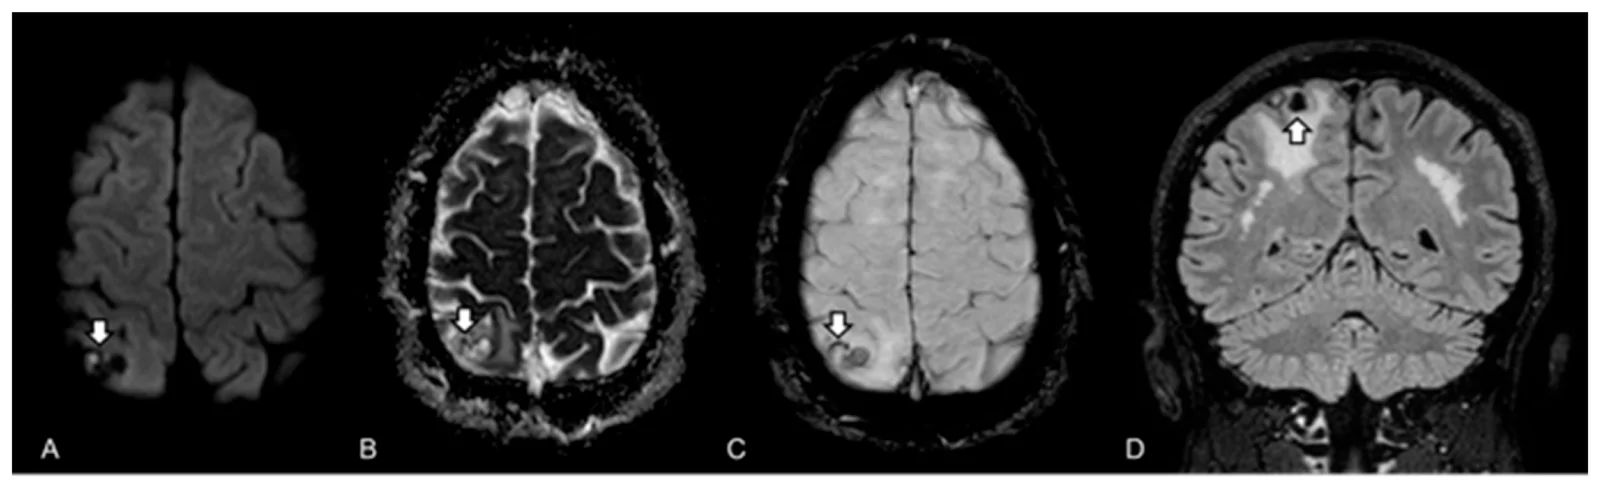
(A) Axial diffusion-weighted image (DWI) demonstrates partial restricted diffusion within the lateral component of the lesion (arrow). (B) Corresponding apparent diffusion coefficient (ADC) map confirms restricted diffusion within this component, with facilitated diffusion medially. (C) Axial susceptibility-weighted image demonstrates peripheral susceptibility predominantly corresponding to the lesion wall (arrow). (D) Coronal FLAIR image demonstrates the lesion (arrow) with extensive surrounding white matter oedema.
